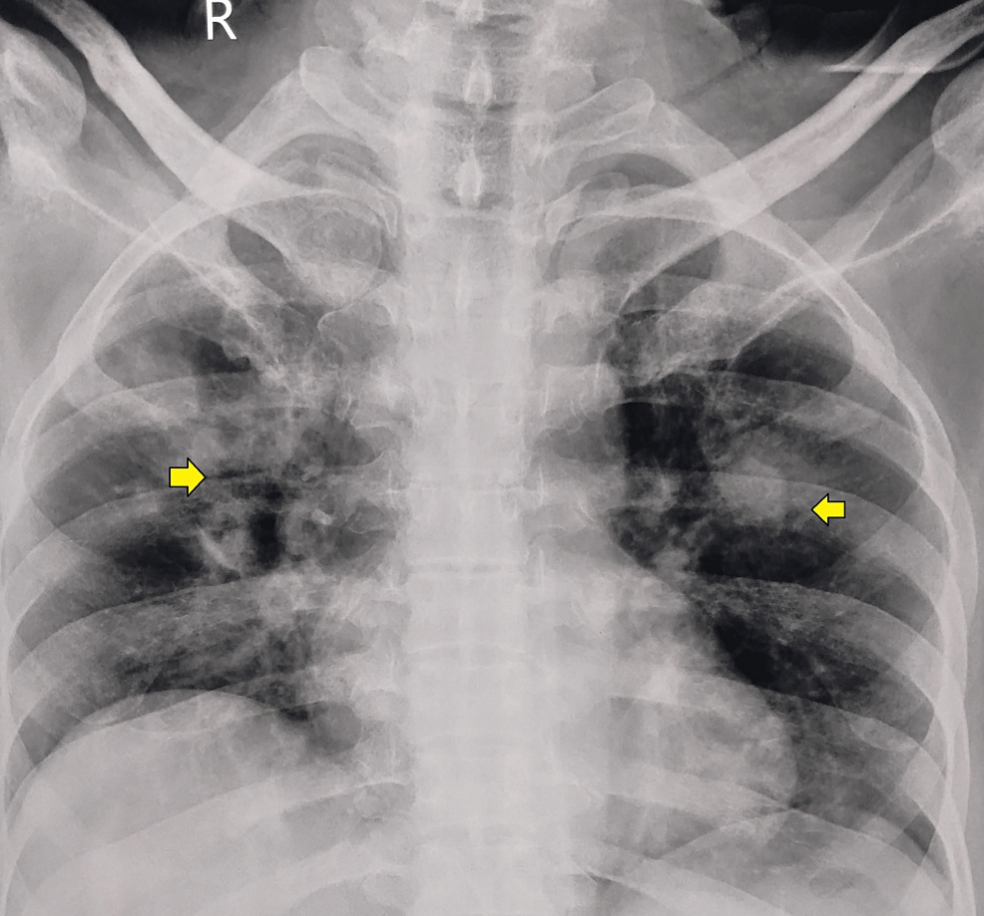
Chest X-ray shows consolidation in both lungs (yellow arrows)
